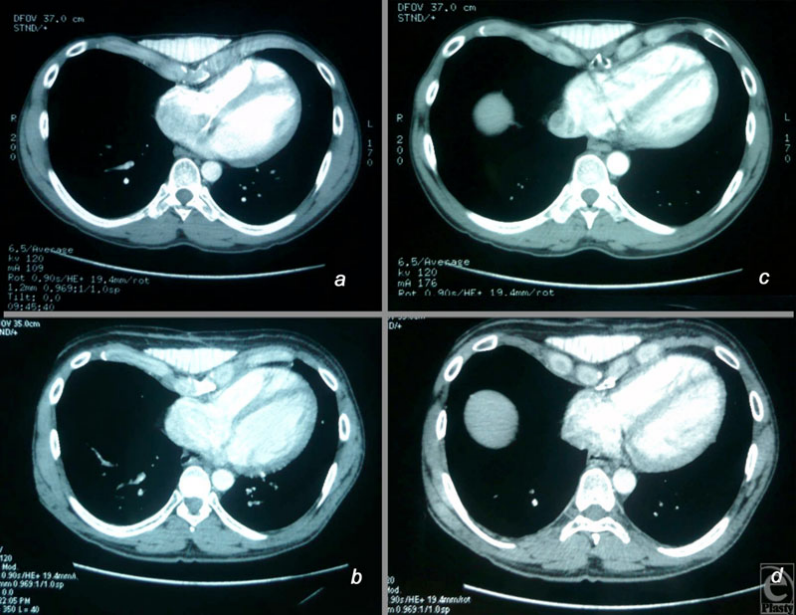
(*a*) and (*c*) Preoperative computed tomographic (CT) scan views showing the thickness over the prothesis before surgery. (*b*) and (*d*) Six months postoperative CT scan views at the same level. On can notice the flaps just over the prothesis.
